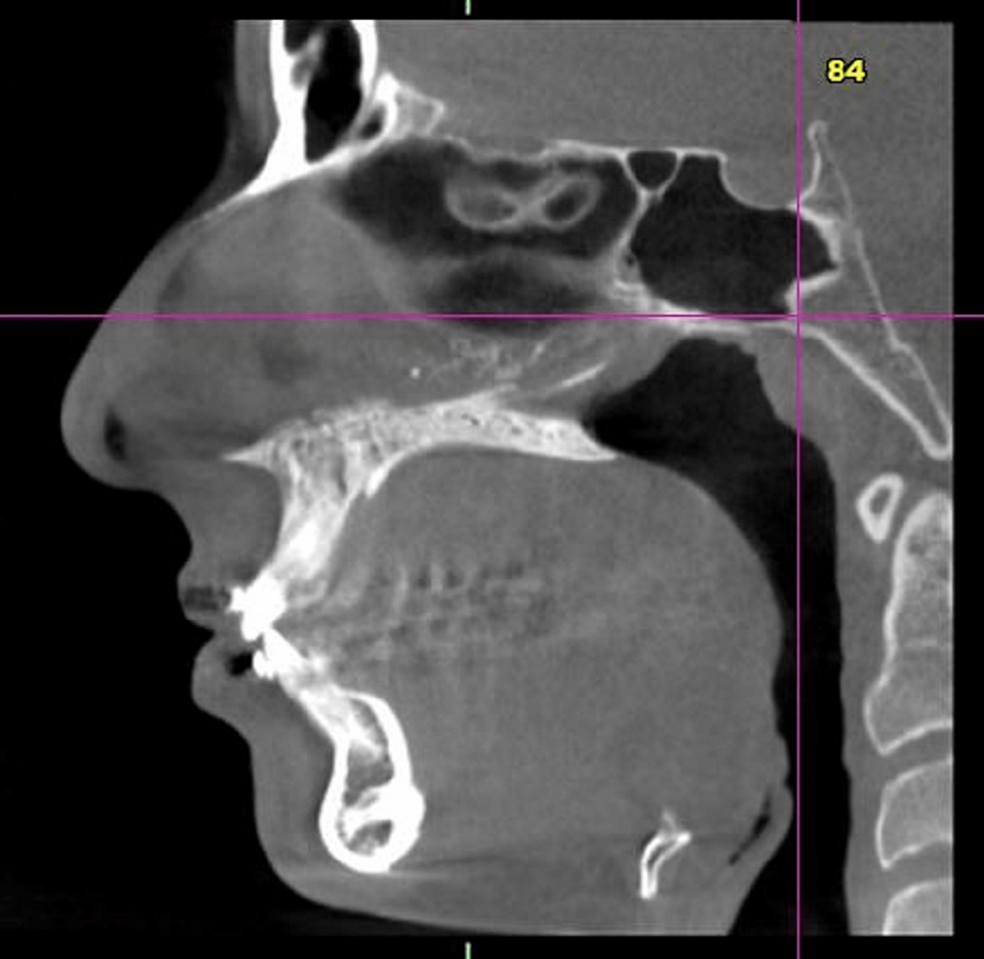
Post-Forsus treatment CBCT CBCT - Cone Beam Computed Tomography
